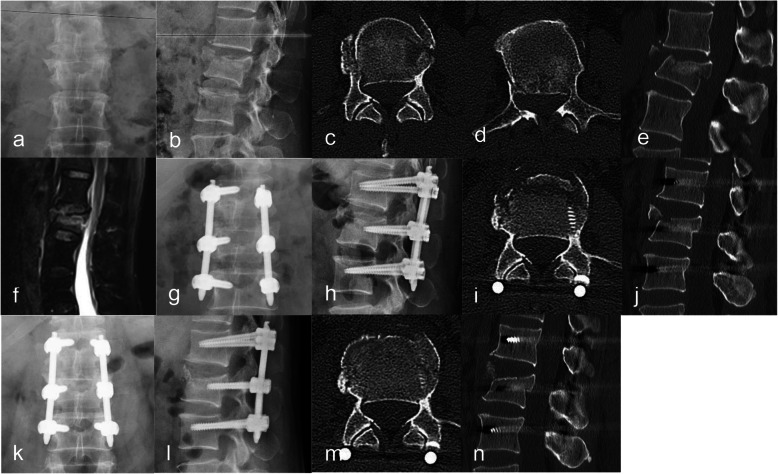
A 42-year-old man was diagnosed with an L2 vertebral burst fracture. a Preoperative anterior and posterior X-ray of the lumbar spine. b Preoperative lateral X-ray of the lumbar spine. c Preoperative CT axial position. d Preoperative CT axial position. e Preoperative CT sagittal position. f Preoperative MRI sagittal position. g Anterior and posterior X-ray of the lumbar spine on 3 days after the operation. h Lateral X-ray of the lumbar spine on 3 days after the operation. i CT axial position on 3 days after the operation. j CT sagittal position on 3 days after the operation. k Anterior and posterior X-ray of the lumbar spine on the last follow-up. l Lateral X-ray of the lumbar spine on the last follow-up. m CT axial position on the last follow-up. n CT sagittal position on the last follow-up
